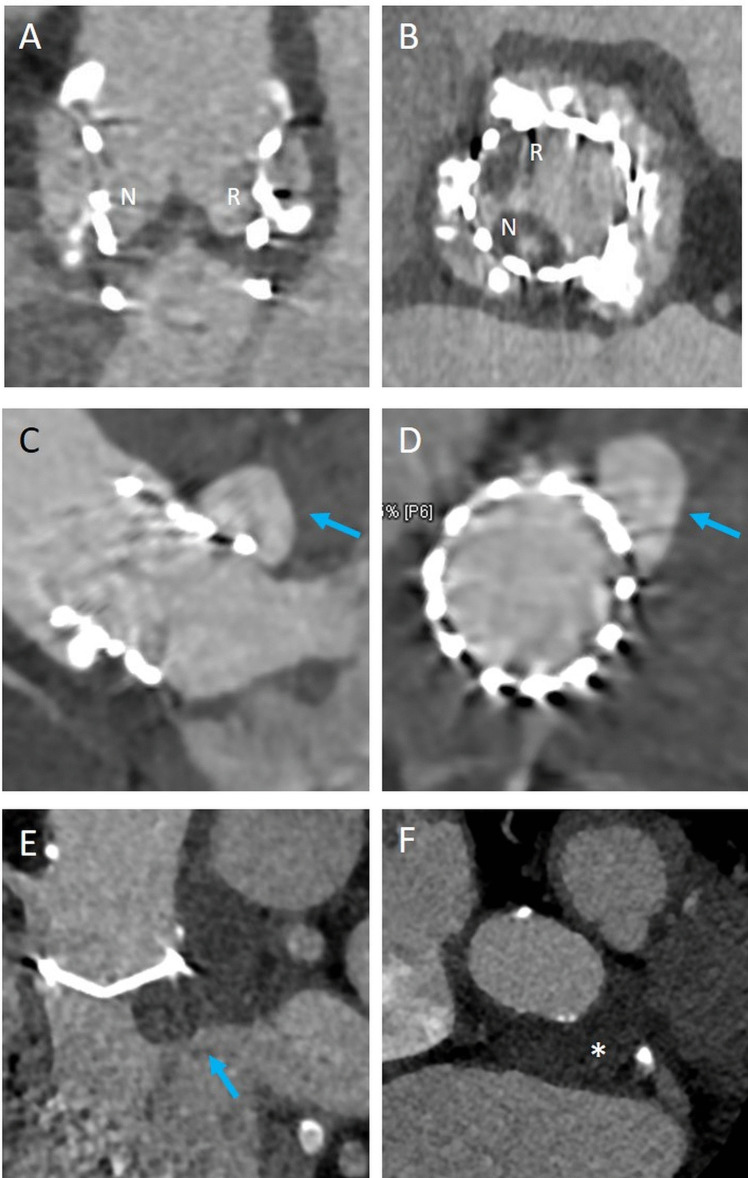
CT for evaluation of valve prosthesis. A, B Hypoattenuated leaflet thickening (HALT) affecting the Neo-NCC (N) and Neo-RCC-leaflet (R) of the TAVI prosthesis. C, D Masked aortic rupture with pericardial effusion (blue arrow; *Courtesy Philipp Breitbart, MD, University Heart Center Bad Krozingen).*
